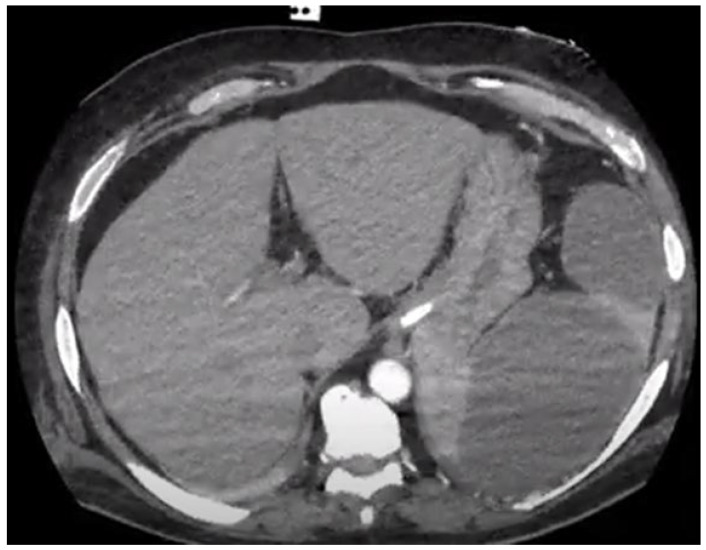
Large splenic abscesses in a patient on chronic haemodialysis with *Enterococcus faecalis* aortic valve endocarditis on non-contrasted CT scan of the abdomen. Source: Instituto Nacional de Cardiologia image collection, 2023.
